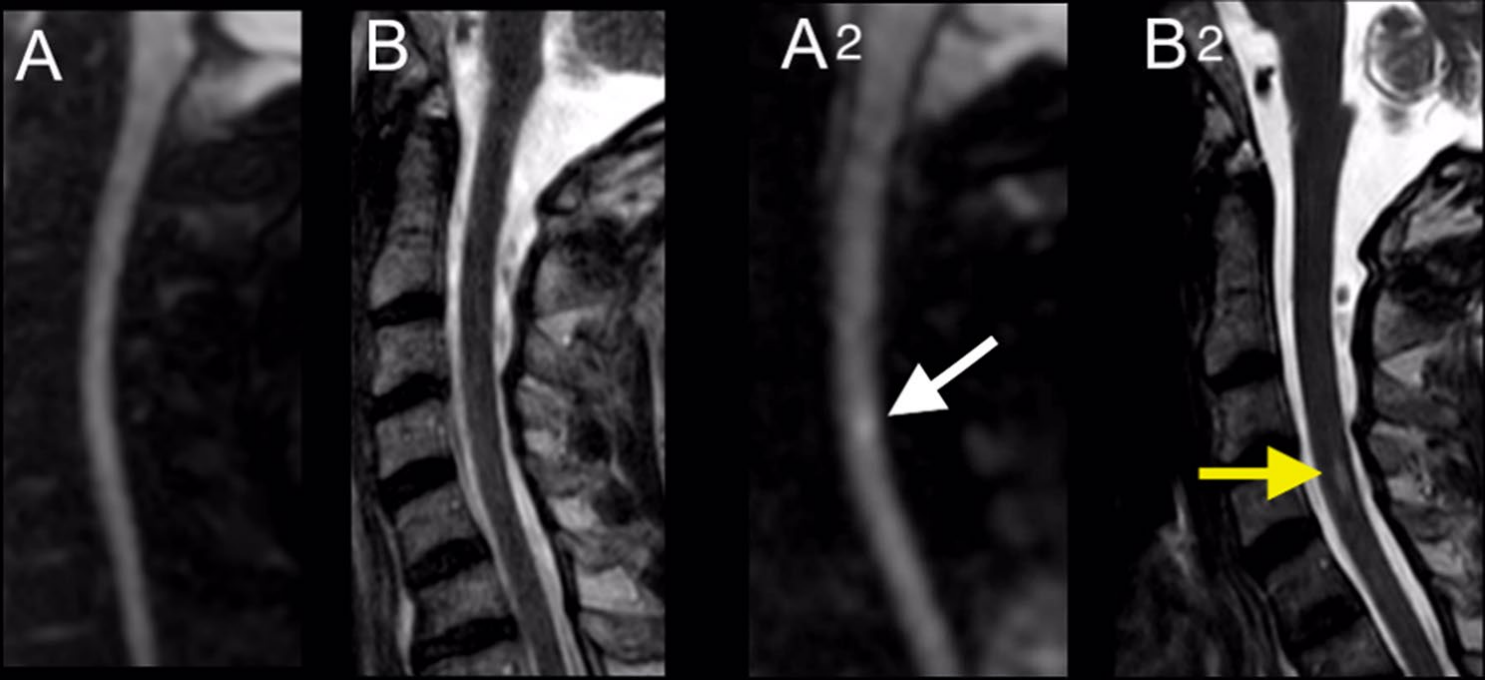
Left: MRI of the cervical spine in sagittal slice orientation. Diffusion-weighted sequence (A), and T2 sequence (B) show no evidence of spinal ischemia. Right: Follow-Up MRI of the cervical spine in sagittal slice orientation. A dot-shaped diffusion restriction (white arrow) is seen in the diffusion-weighted sequences (A2) with a hyperintense correlate (yellow arrow) in the T2 sequence (B2) at the level of the fourth cervical vertebral body, consistent with spinal ischemia
